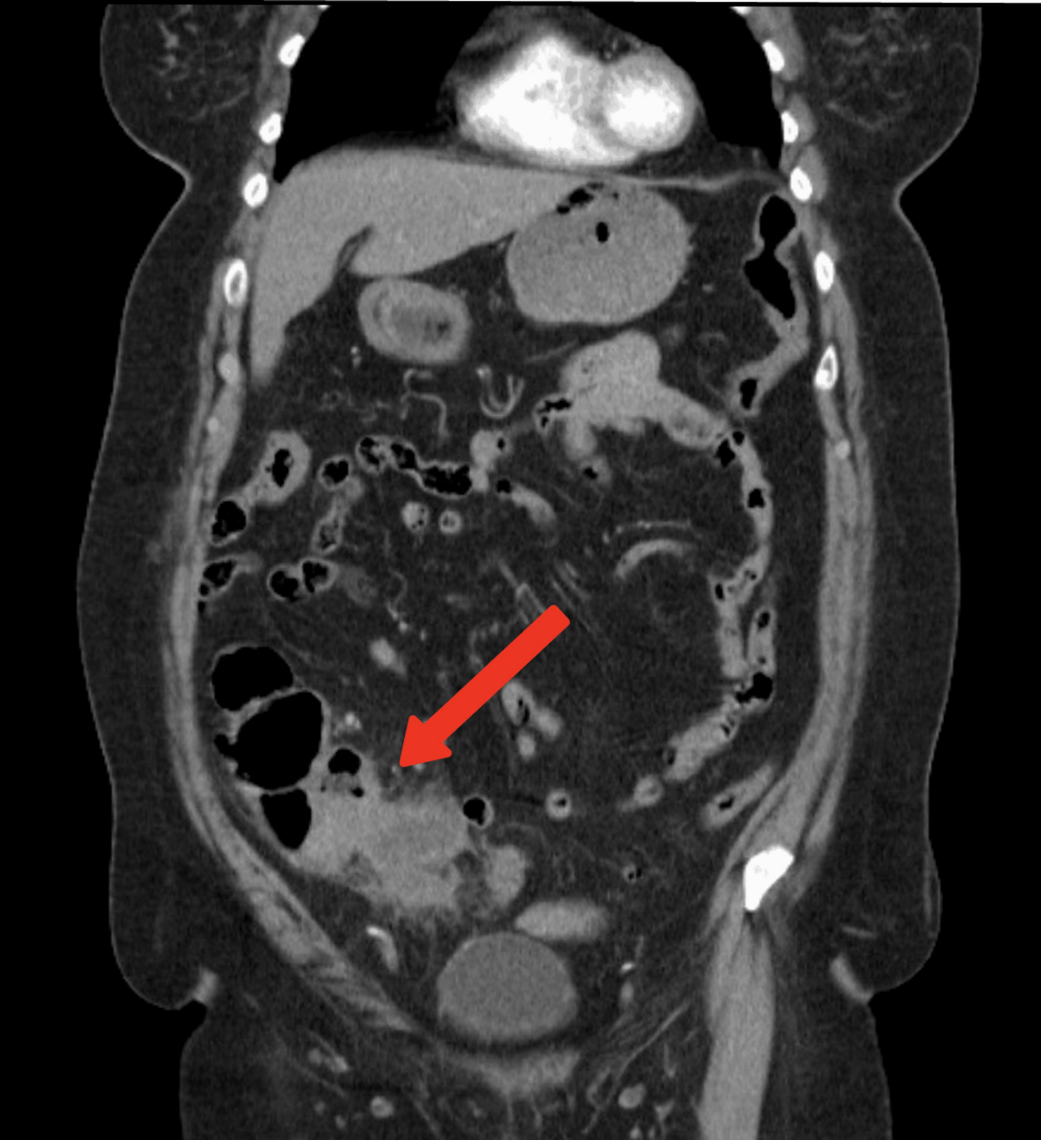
CT scan of abdomen and pelvis - coronal view
